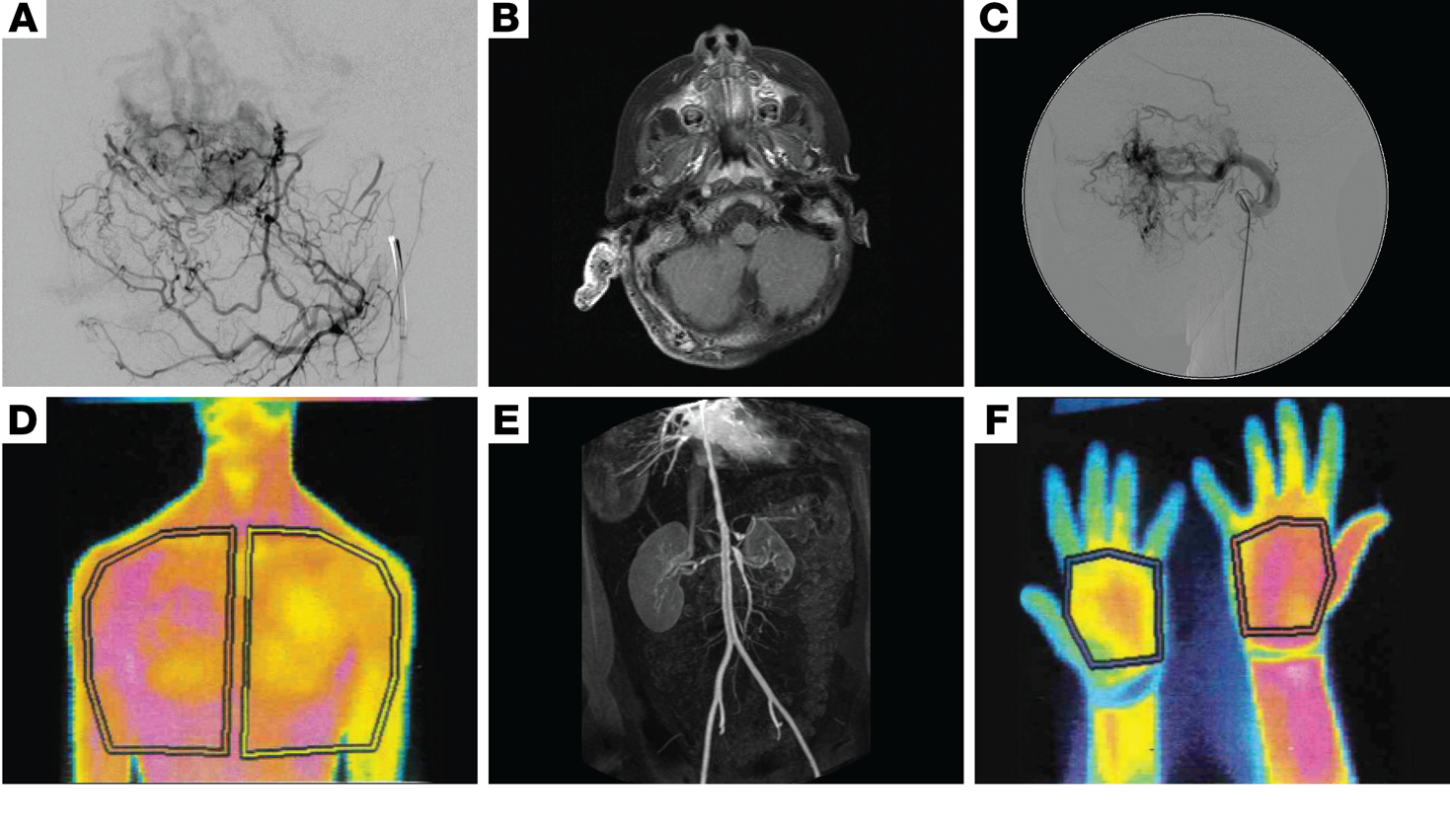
Imaging of sporadic VMs secondary to mutations in MAPK pathway genes demonstrating involvement of all blood vessel sizes. (A) Lateral image from a digitally subtracted angiogram showing a leash of small, abnormal vessels shunting through a dense capillary bed to early filling veins. (B) Axial contrast-enhanced fat-saturated T1 weighted MRI image showing a grossly enlarged right pinna and thickened posterior auricular soft tissues. The abnormal tissue is filled with multiple signal voids, representing enlarged abnormal vessels. The pinna enhances avidly. (C) Lateral image from a digitally subtracted angiogram, with the catheter tip in the grossly enlarged left internal maxillary artery, which supplies a leash of abnormal high-flow vessels in the face. (D) Thermography of low-flow VMs with overgrowth of the left chest wall and arm demonstrates increased temperature (shown in magenta) compared with the right-sided structures (E), and in the right forearm and thumb compared with the left (F). 3D reconstruction of an abdominal CT angiogram demonstrating multifocal vascular disease, with severe stenoses of the descending aorta, coeliac axis, superior mesenteric artery origin, and right renal artery (E).
